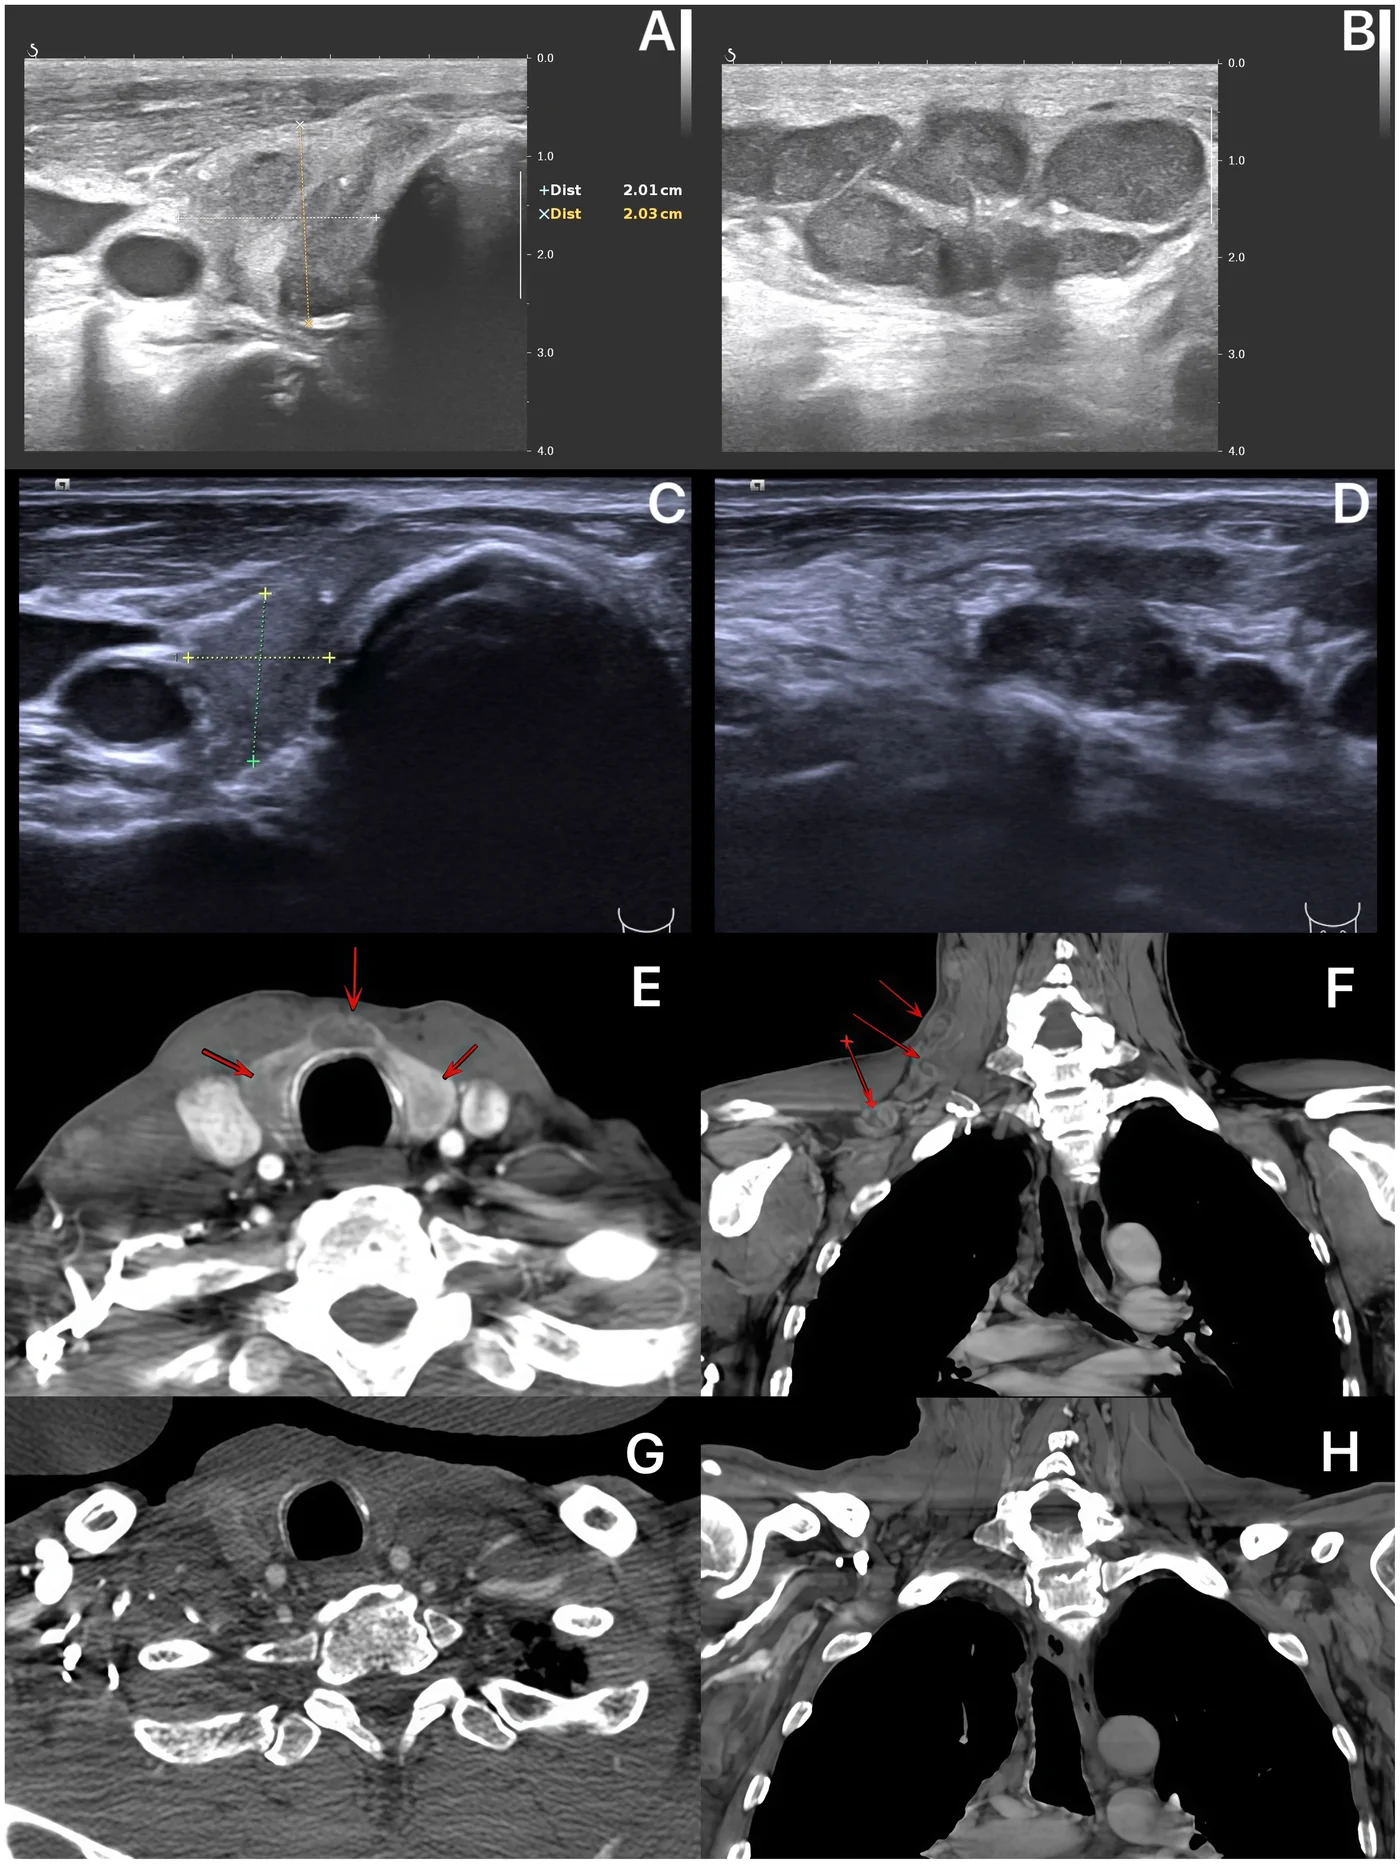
(A,B) Pre-treatment ultrasound of thyroid nodules and enlarged lymph nodes. (C,D) Post-treatment ultrasound of the thyroid and enlarged lymph nodes. (E,F) Pre-treatment neck/chest enhanced CT of thyroid nodules and enlarged lymph nodes. (G,H) Post-treatment neck/chest enhanced CT of the thyroid and enlarged lymph nodes.
